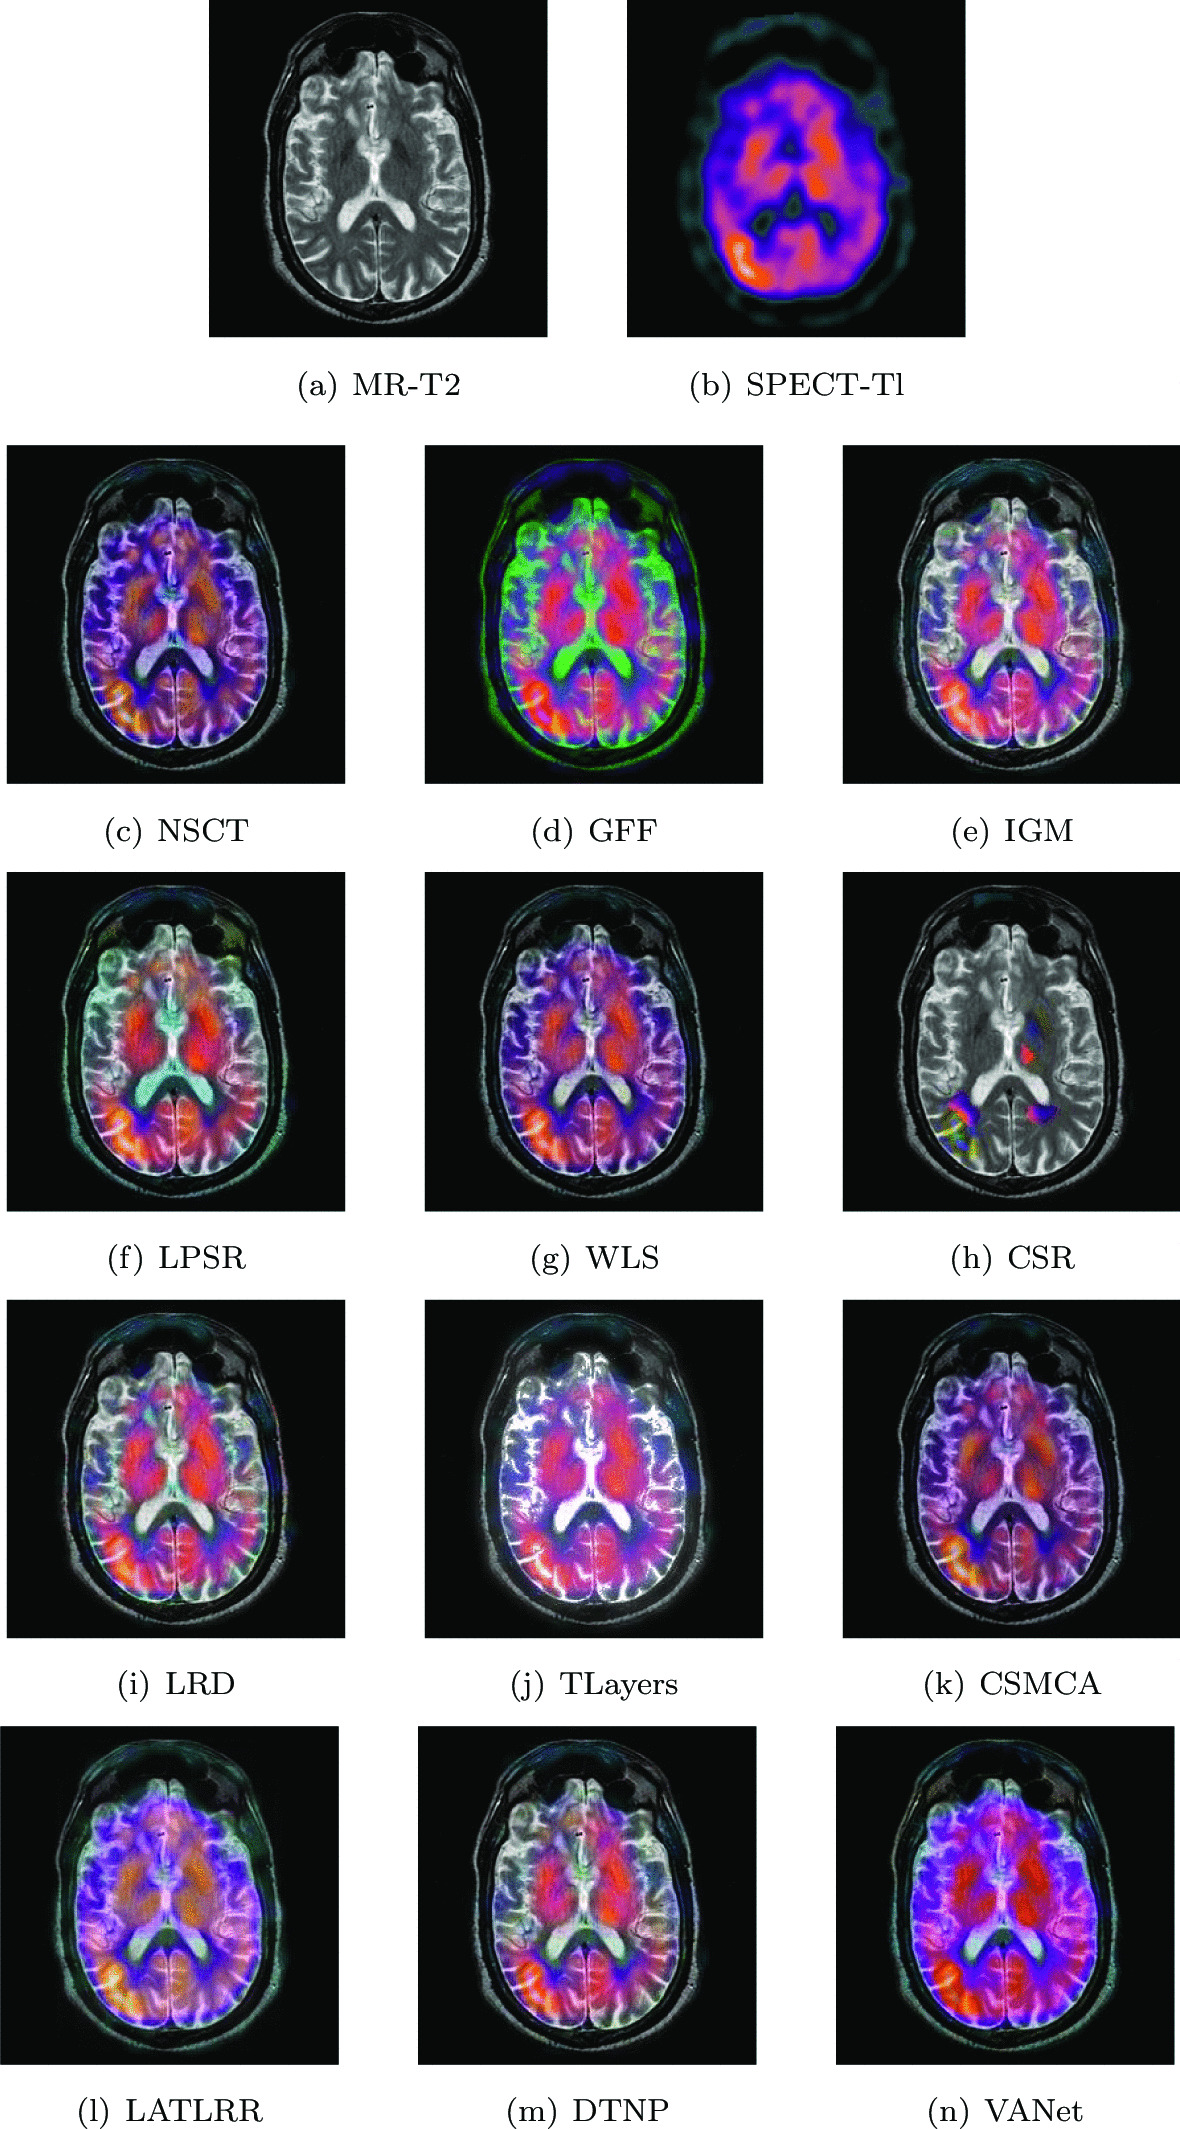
The first set of fused MRI-SPECT images from 9 methods on subacute stroke
